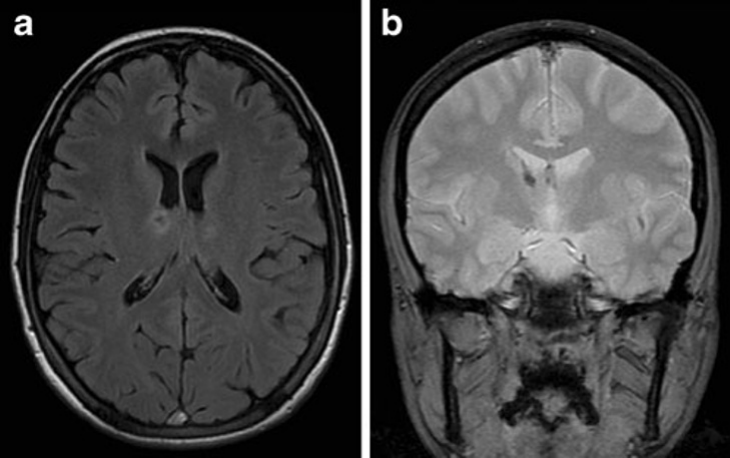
Acute hemorrhagic infarction in the right thalamus due to venous sinus occlusion in a 30-year-old woman with a history of hormonal contraception. a FLAIR image and b gradient-echo T2*-weighted image proving hemorrhagic transformation. FLAIR, fluid-attenuated inversion recovery
